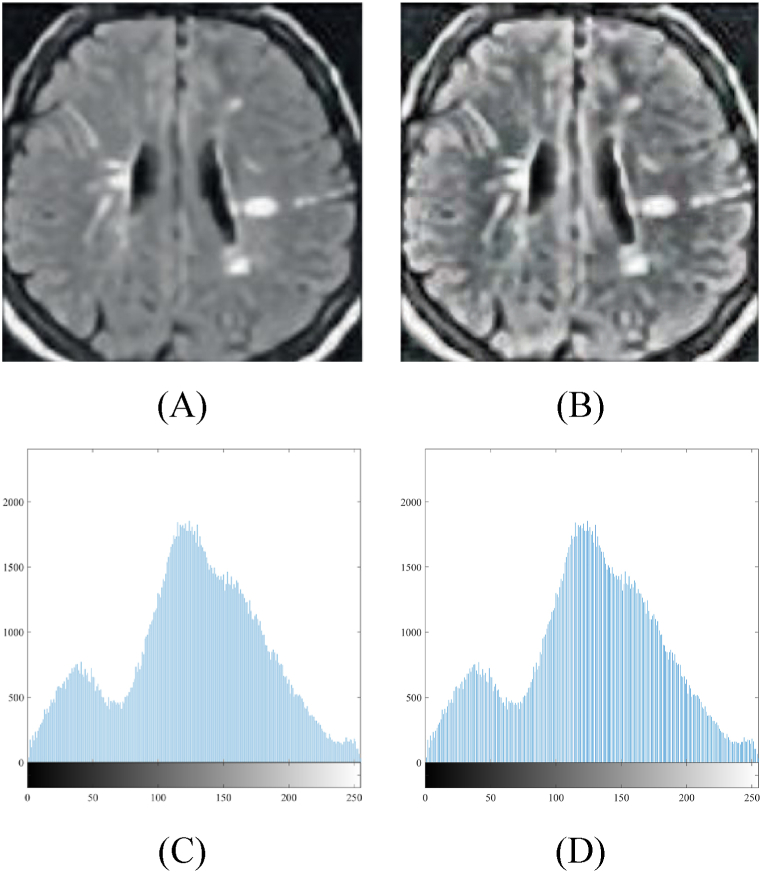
CLAHE applied to Brain tumor MRI: (A) input image; (B) image after CLAHE; (C) histogram of (A); (D) histogram of (B).
